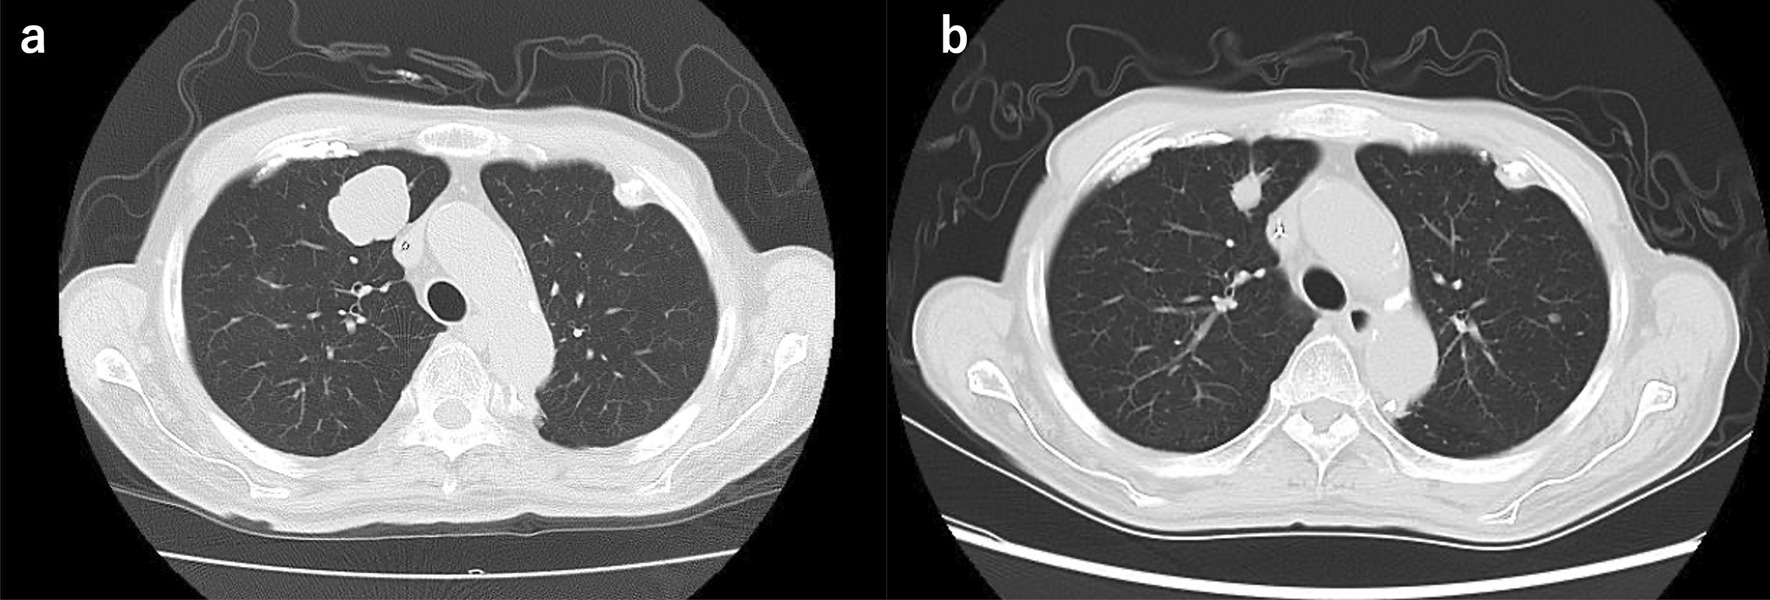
Chest computed tomography findings. Multiple lung metastases in the right upper lobe. a Postoperative month 21. b Postoperative month 29
